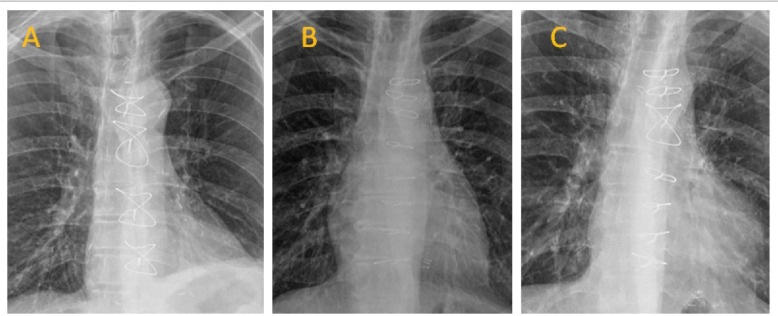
Chest radiographs of sternal closure techniques. A) Figure-of-8; B)
